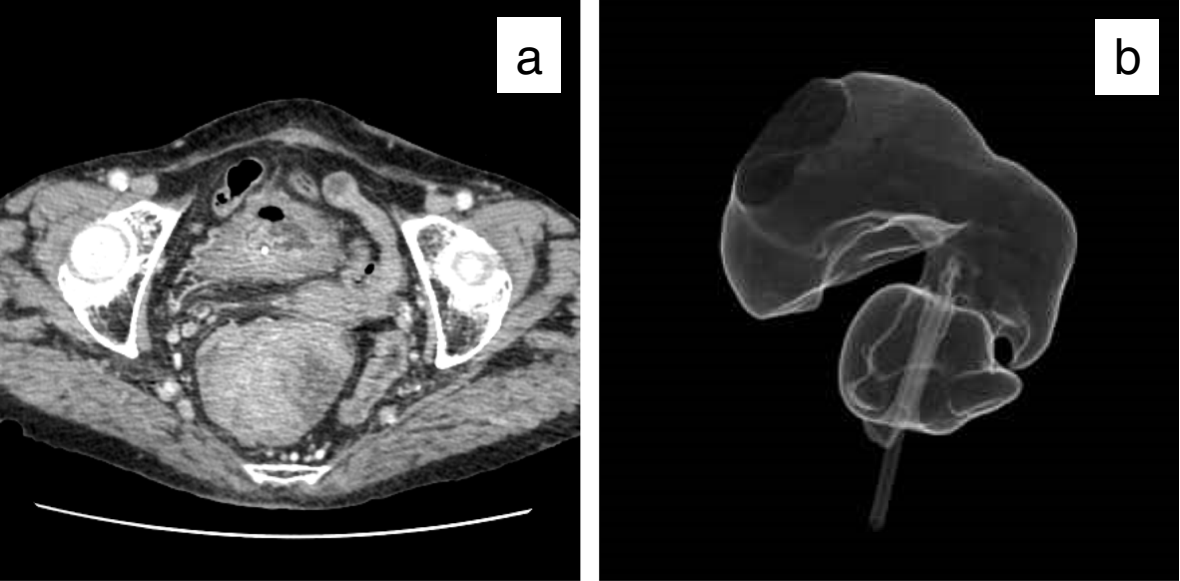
Computed tomography findings. a Enhanced tumor was found in the lower rectum without lymph node metastasis. b The computed tomography colonography showed that the tumor extended into anal canal
